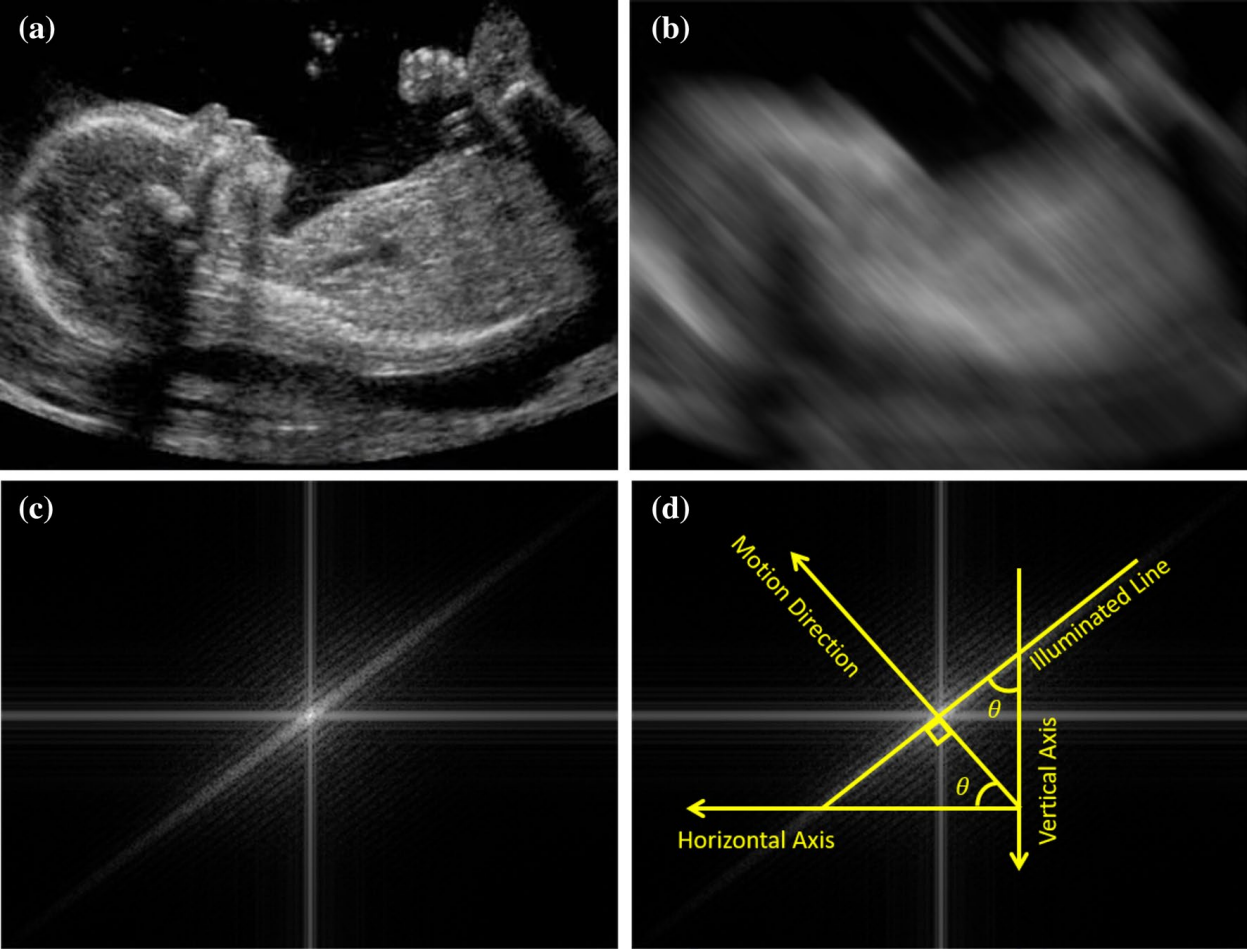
(a) Fetus ultrasound image, (b) the blurred version of image generated by linear motion, (c) Fourier transform of image (b), (d) the geometrical representation of blur angle $$\theta $$ and linear motion direction shown in (c).
